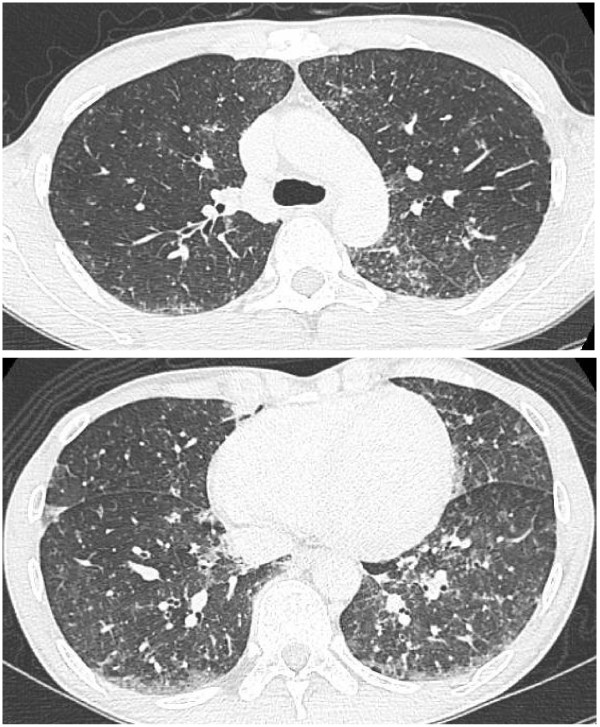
High-resolution chest computed tomography (CT) scan taken at our patient’s second admission. The CT scan shows diffuse, randomly distributed, ill-defined, centrilobular micronodules with patchy ground glass opacity in both lung fields.
